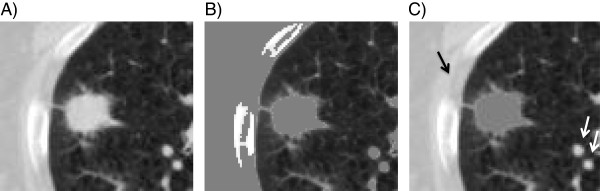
Method to evaluate tumor volume using an image analyzing system, Dr. View/LINAX. (A) First, the CT images were displayed at an optimal window level of -400 HU with a width of 1,400 HU. (B) The region above -250 HU was automatically extracted (gray areas). (C) The gray-painted structures outside the tumors such as vessels and chest walls (arrows) were manually excluded, and the tumor volume of the gray-painted regions was calculated.
